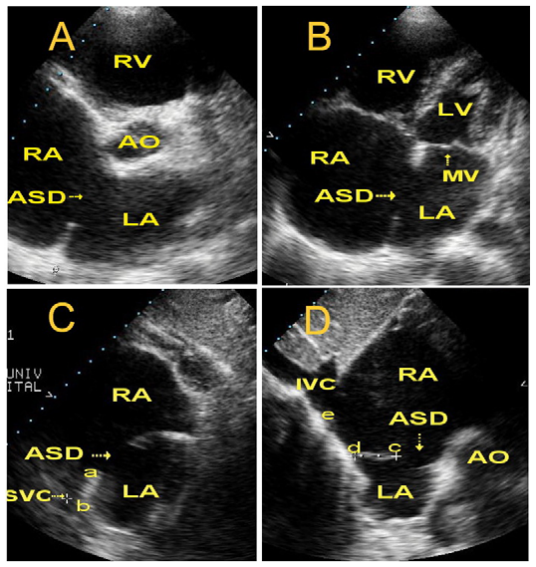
Measurement of ASD diameter and surrounding rims using TTE in different cross sections in a patient with ASD and a deficient superior-anterior rim. (A) Parasternal short-axis view showing measurement of the ASD diameter and the length of superior-posterior rim. The superior-anterior rim was absent. (B) Apical four chamber view showing measurement of the ASD diameter and the rim length from ASD to the mitral annulus and to the top of the atrium. (C and D) Subcostal view showing measurement of the ASD diameter, total atrial septal length, and rim length from the ASD to the mitral annulus and the superior vena cava (SVC) and inferior vena cava (IVC) entrances. Distance from “a” to “b” is the rim length of the SVC side, “c” to “d” rather than “c” to “e” is the rim length of the IVC side. The distance from “d” to “e” is the posteroinferior wall of the right atrium. ASD = atrial septal defect, AO = ascending aorta, LA = left atrium, RA = right atrium, LV = left ventricle, RV = right ventricle, IVC = inferior vena cava, SVC = superior vena cava.
